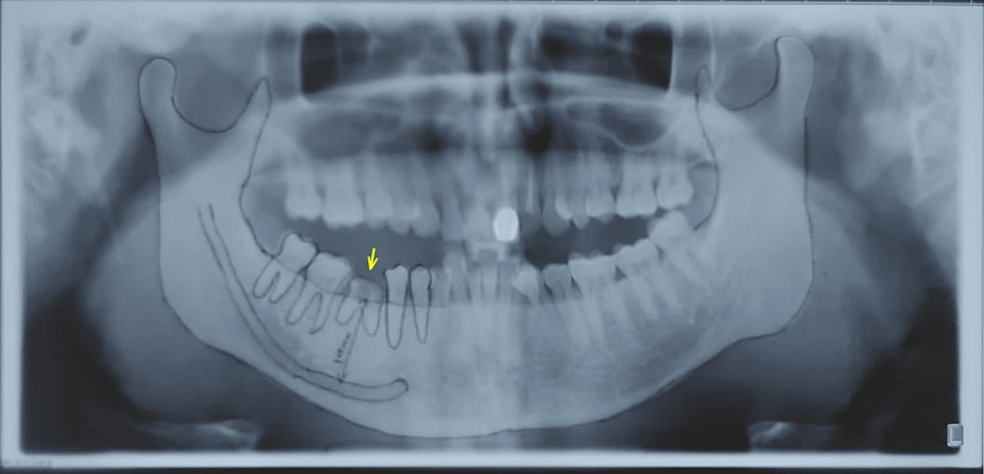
Preoperative orthopantomogram with the yellow arrow showing infected root stump in 46 regions (right mandibular first molar)
